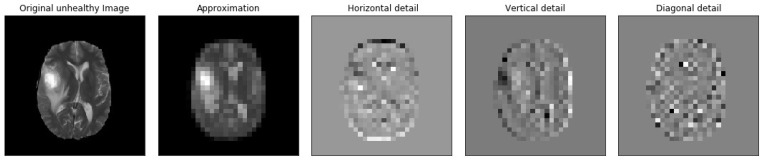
Samples of our Level 3 DWT decomposition of a non-healthy MR image type T2.
